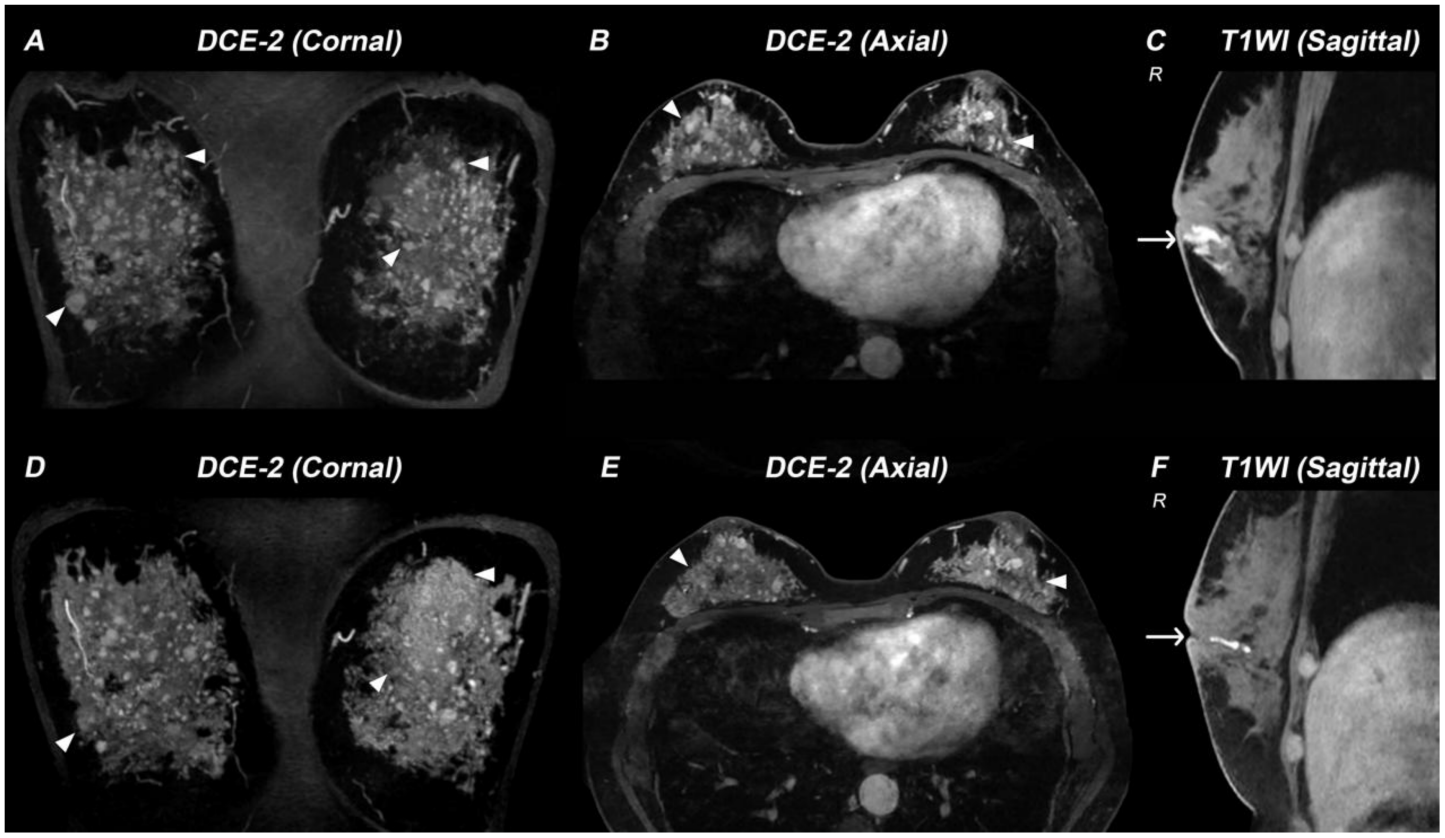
Breast MRI demonstrating therapy-related changes in a patient with 17α-hydroxylase/17,20-lyase deficiency (17-OHD). (A–C) MR images acquired before 17-OHD therapy: DCE-2 MRI (A, B) images and T1WI image (C) show multiple nodules (triangle) and enlarged mammary ducts (arrow) in the breasts of this case. (D–F) MR images acquired six months after 17-OHD therapy: DCE-2 MRI (D, E) and T1WI image (F) show significant reduction of nodules (triangle) and mammary ducts (arrow).
